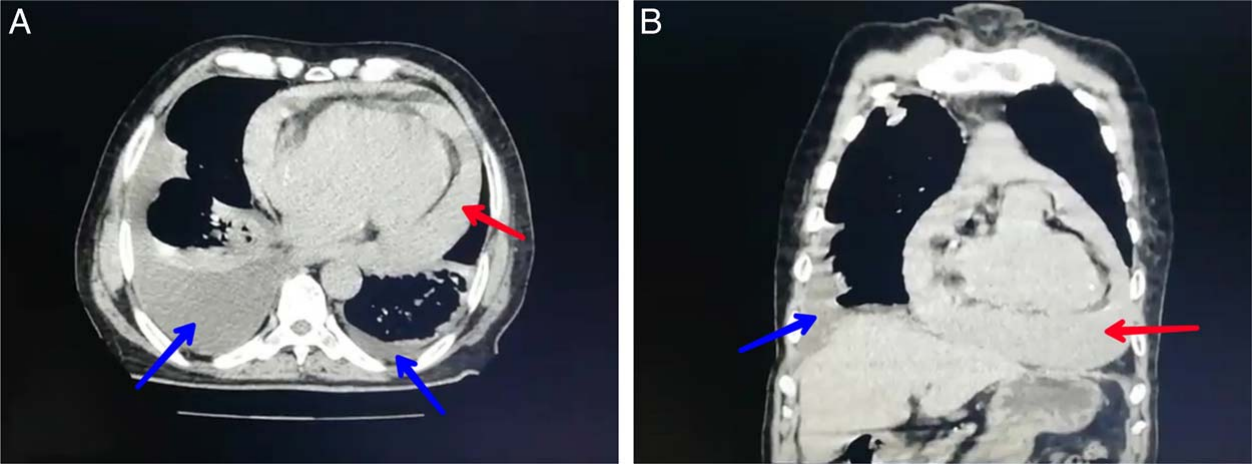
CT (A) shows a cross section, while (B) shows a coronal section. Both sections detect a pleural effusion (indicated by the blue arrow) and a pericardial effusion (indicated by the red arrow).
